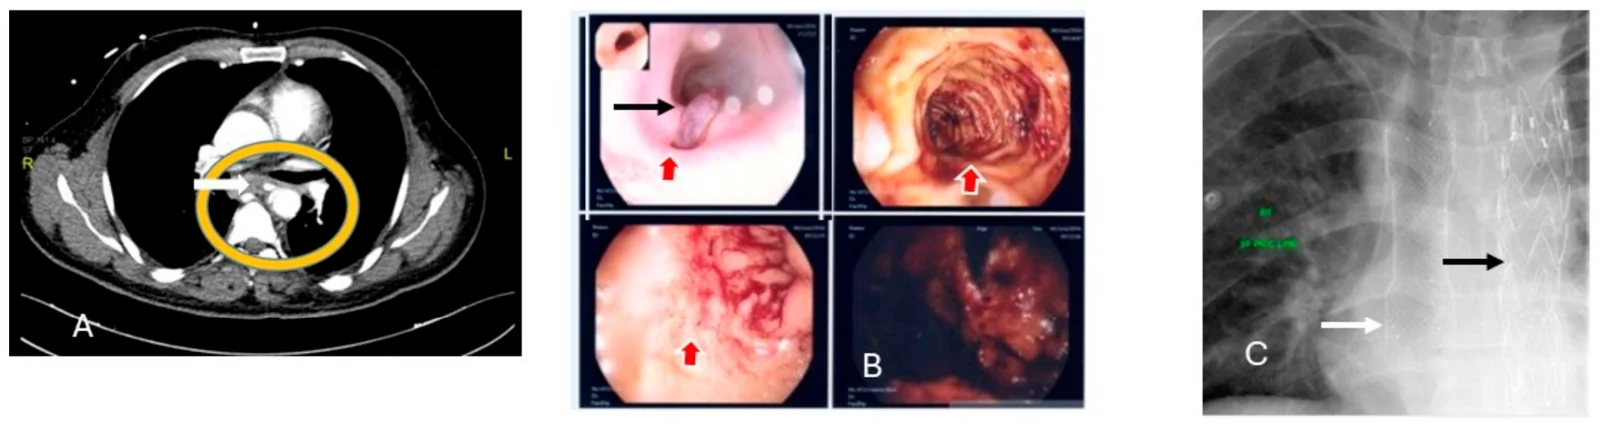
(Case 3): (A) CT scan with contrast, showing large contrast extravasation into the esophagus, indicating AEF (circle and white arrow). (B) Picture taken for upper endoscopy. In the lower esophagus, it shows a clot protruding (black arrow) through an ulcer (red arrow) and pointing down towards the distal esophagus. The other pictures of the stomach show blood within the stomach (red arrow) and duodenum (red arrow). (C) Chest X-ray showing the EVAR stent (black arrow) and esophageal stent (white arrow).
